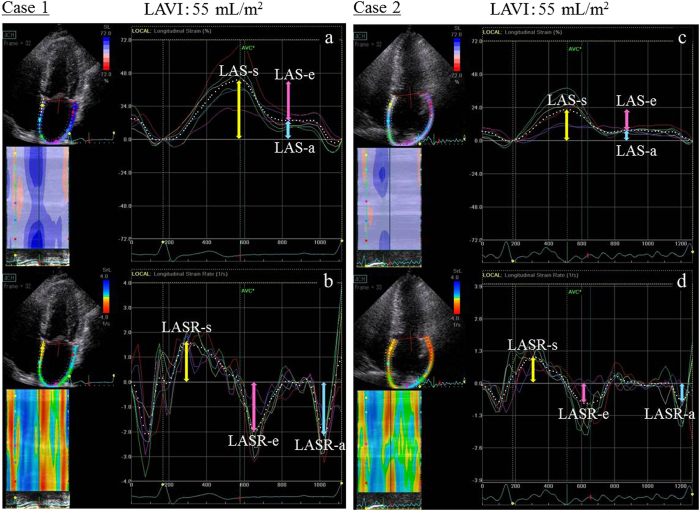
Although cases 1 and 2 had a similar left atrial volume index (LAVI, approximately 55 mL/m^2^), LA function, as indicated by 2D speckle-tracking echocardiography, was significantly different. In case 1, reservoir (r), conduit (e), and contractile (a) strains and strain rates were 41.1%, −25.6%, and −15.5%, and 1.68 s^−1^, −2.0 s^−1^, and −2.2 s^−1^, respectively. On the other hand, case 2 had lower reservoir, conduit, and contractile strains and strain rates (22.1%, −13.9%, and −8.2%, and 1.02 s^−1^, −0.70 s^−1^, and −0.82 s^−1^, respectively). Abbreviations: LAS, left atrial strain; LASR, left atrial strain rate.
